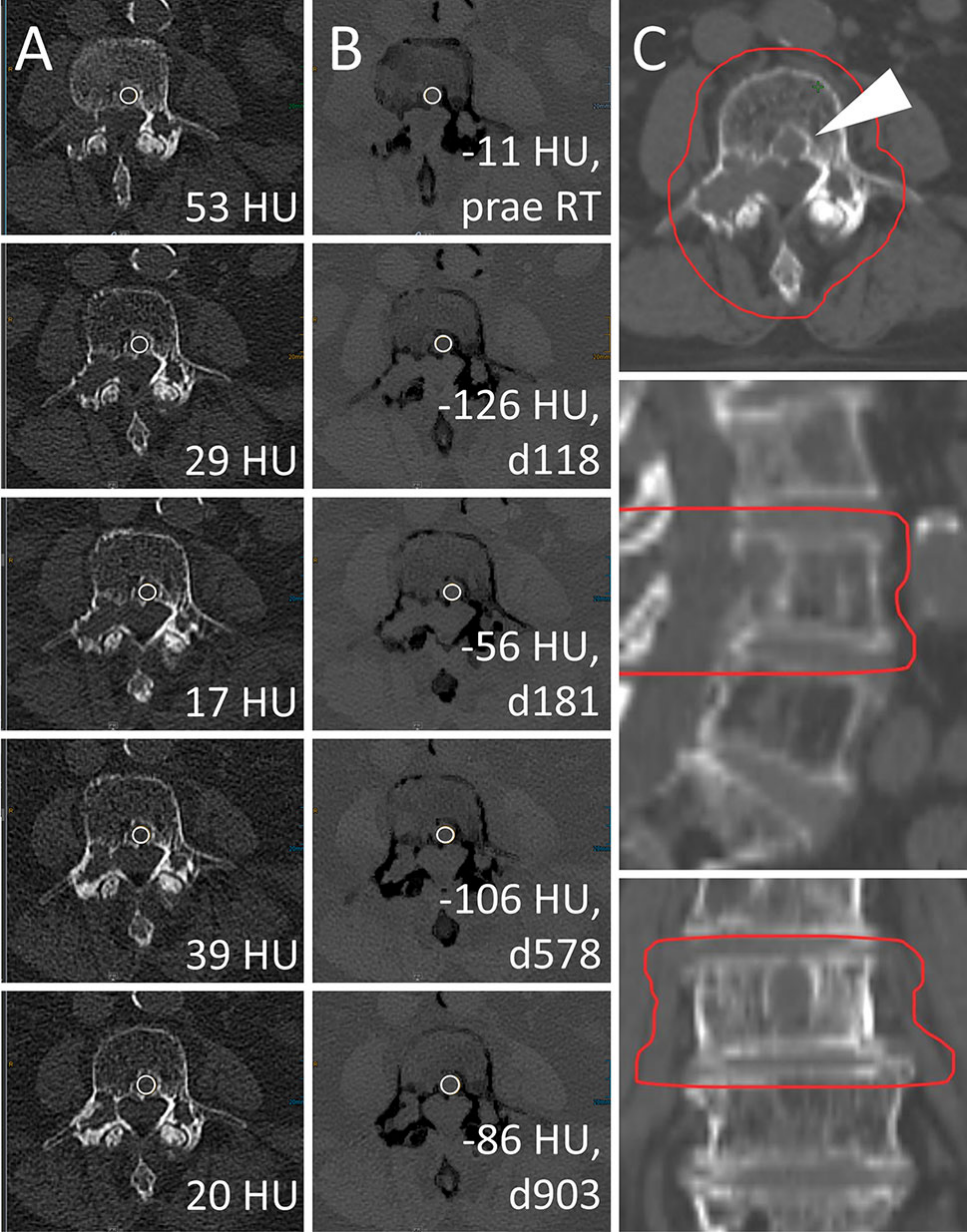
Radiotherapy (RT) response of a lytic bone lesion with high calcium content. Male, 70 years old (at time of RT) patient with history of multiple myeloma (MM). Total radiation dose was 30 Gy (3 x 10 Gy); RT planning CT is shown in column (C). Axial CT slices as conventional images [column (A)] and virtual noncalcium (VNCa) post-processed images [column (B)] before RT (first row) and 118, 181, 578, and 903 days after RT (second to fifth row, respectively). On VNCa images, irradiation response in the assessed target lesion (white arrowhead) is apparent by decreasing, fatty attenuation values. Bone mineral content is estimated by subtraction of conventional and VNCa attenuation to assess lesion calcification as another sign of therapy response. In this case, the calcium specific attenuation portion increases from 64 HU prior to RT (*att*
